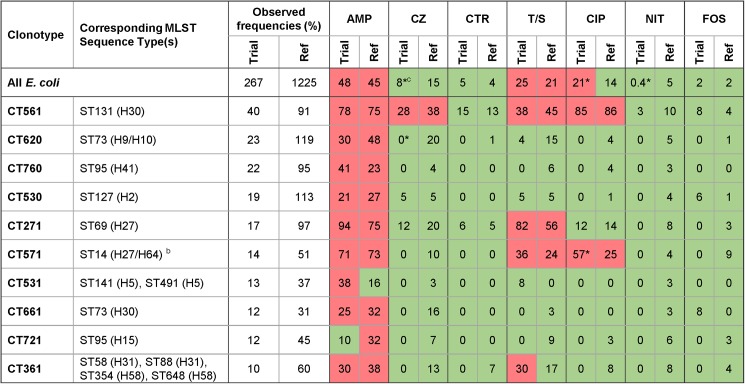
Comparison of antibiotic resistance prevalence among study vs. local reference *Escherichia coli* isolates for the 10 most frequent study clonotypes. ^a^ For each CT (clonotype) the most prevalent (≥ 90% of isolates) sequence type (ST), as determined by multilocus sequence typing, is shown, with its subclone determined by CH typing (H) in parentheses, where applicable. For CTs represented by multiple STs (each accounting for ≤ 90% of the constituent isolates) up to four major STs and the corresponding H subclones are listed. ^b^ CT571 comprises ST14 (H27), ST404 (H27) and ST1193 (H64), which all belong to clonal complex of ST14. ^c^ For the study set and local reference set (‘Ref’), antibiotic resistance prevalence for all *E*. *coli* and for major clonotypes is given as percent of resistant isolates in relation to the total number of isolates, overall or within the clonotype; green color indicates resistance below conventional threshold (≤ 20%), red color–above it (> 20%); the resistance within study and reference sets was compared individually for each antibiotic using a two-sided Fisher’s exact test; * P < .05.
